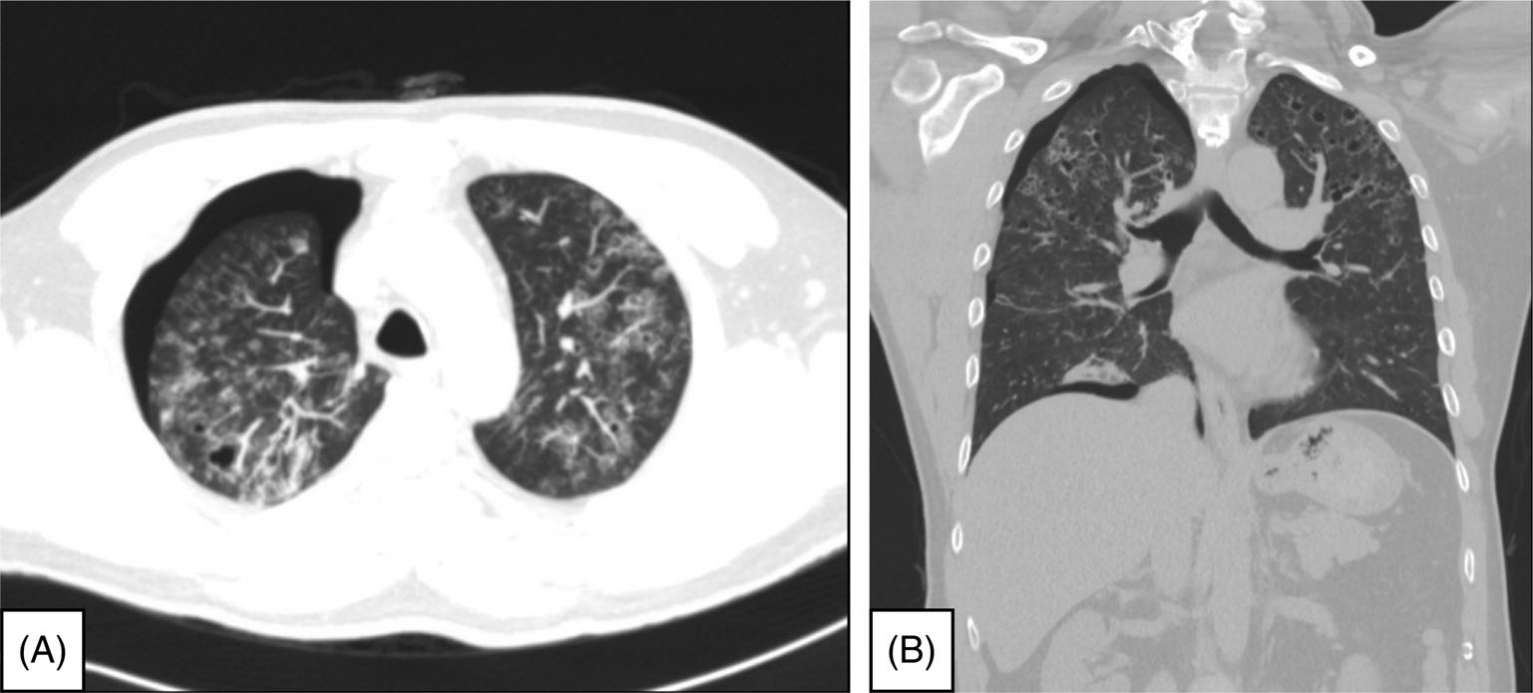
(A) Transverse view and (B) coronal view of the lung window of chest computed tomography. Right spontaneous pneumothorax and lung atelectasis. Bilateral multiple bizarre‐shaped, thick‐walled, ill‐defined, air‐density infiltrated parenchyma cysts; increased consolidations; and multiple ground‐glass cavitary nodules with interstitial thickening were noted
